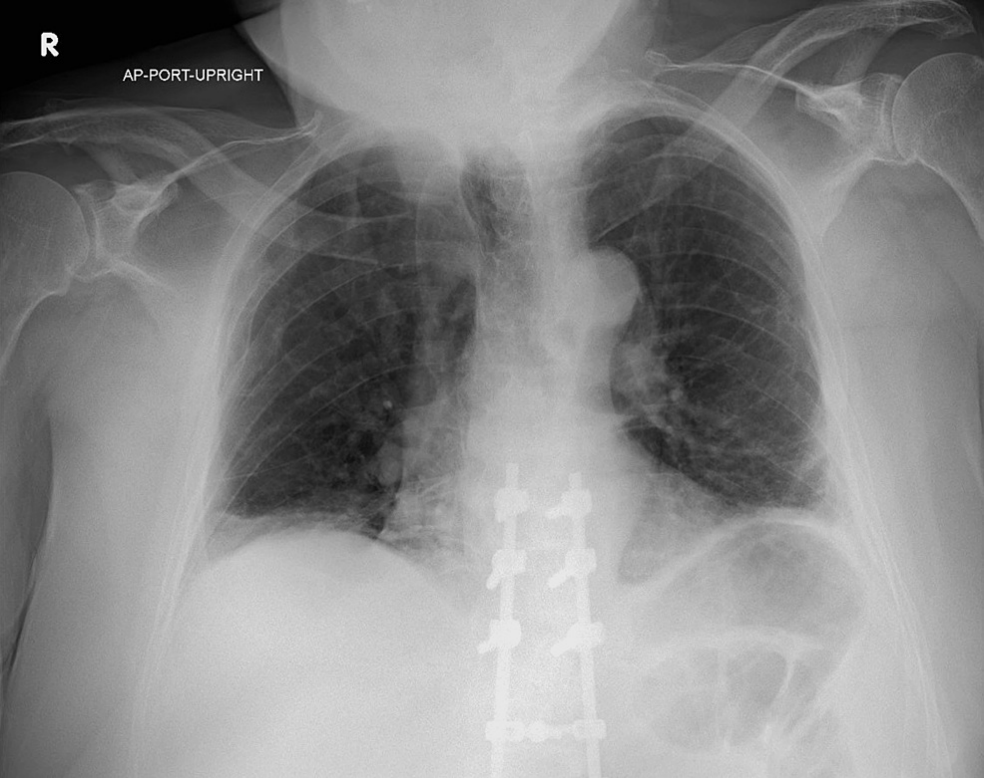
CXR on admission CXR on admission showed predominantly linear bibasilar opacities, low lung volumes, and subtle consolidations. The enlargement of the cardiopericardial silhouette was similar to prior imaging. It also shows partially imaged thoracolumbar posterior spinal fusion hardware and multiple remote bilateral rib fractures, both from prior motor vehicle collisions. CXR, chest x-ray
